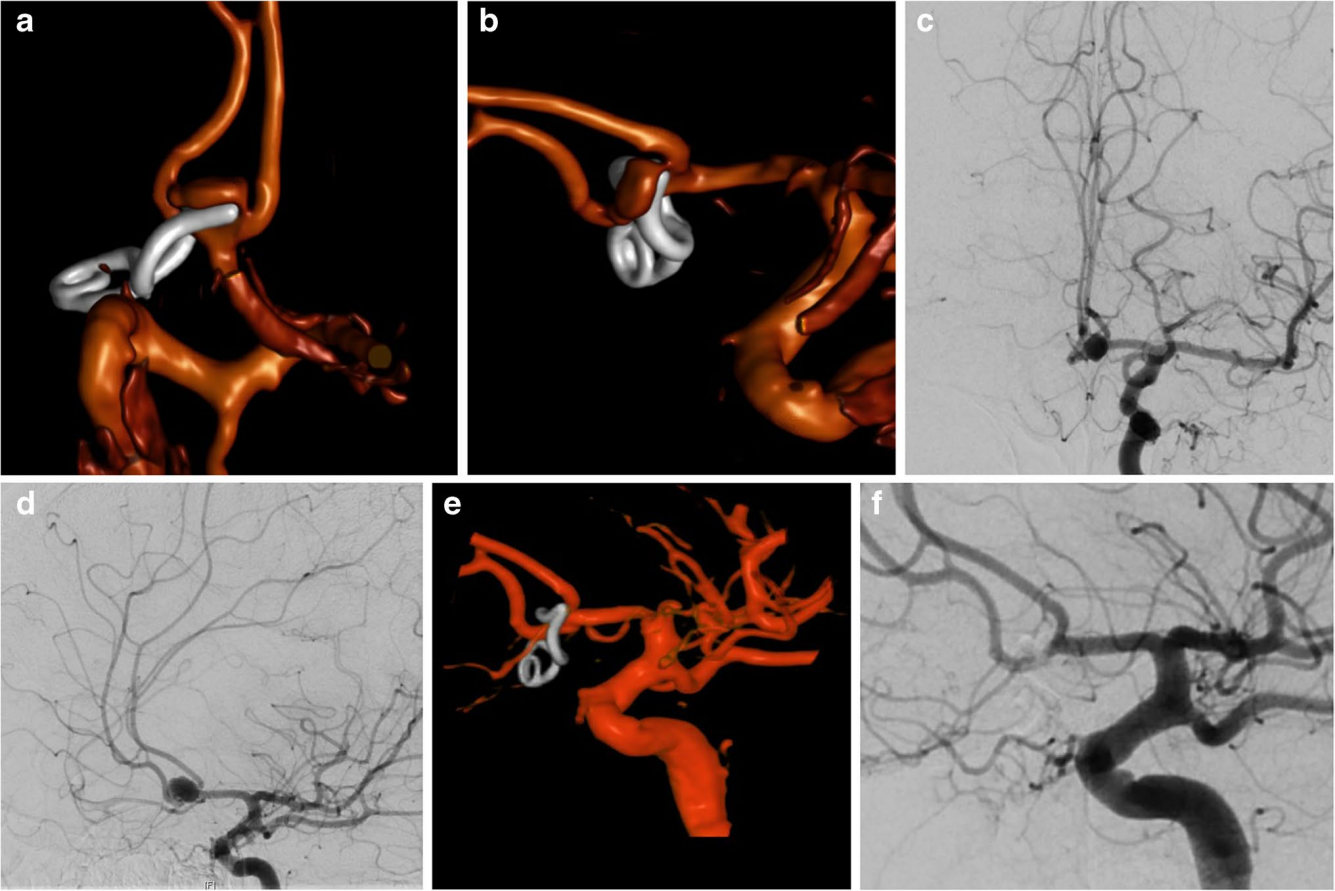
Clipped aneurysm showing neck remnant. Postoperative 3D reconstruction of CTA showing suspicion of a small neck remnant: A AP projection, B lateral projection, which was then confirmed by conventional angiography, C AP projection, D oblique projection. The remnant was subsequently coiled; E 3D reconstruction, F oblique projection, achieving complete occlusion
